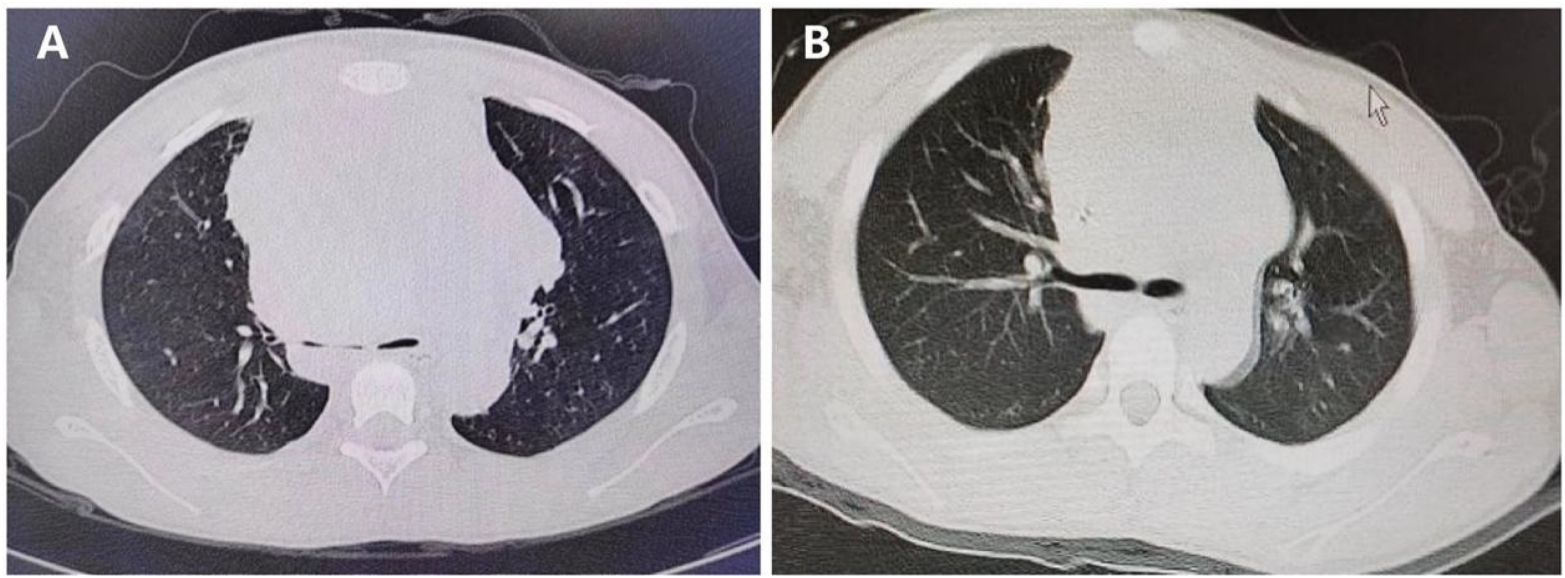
Lung CT before and after steroid Use. (A) Severe compression of the main bronchi before steroid use. (B) Reduction in bronchial compression after seven days of steroid therapy.
